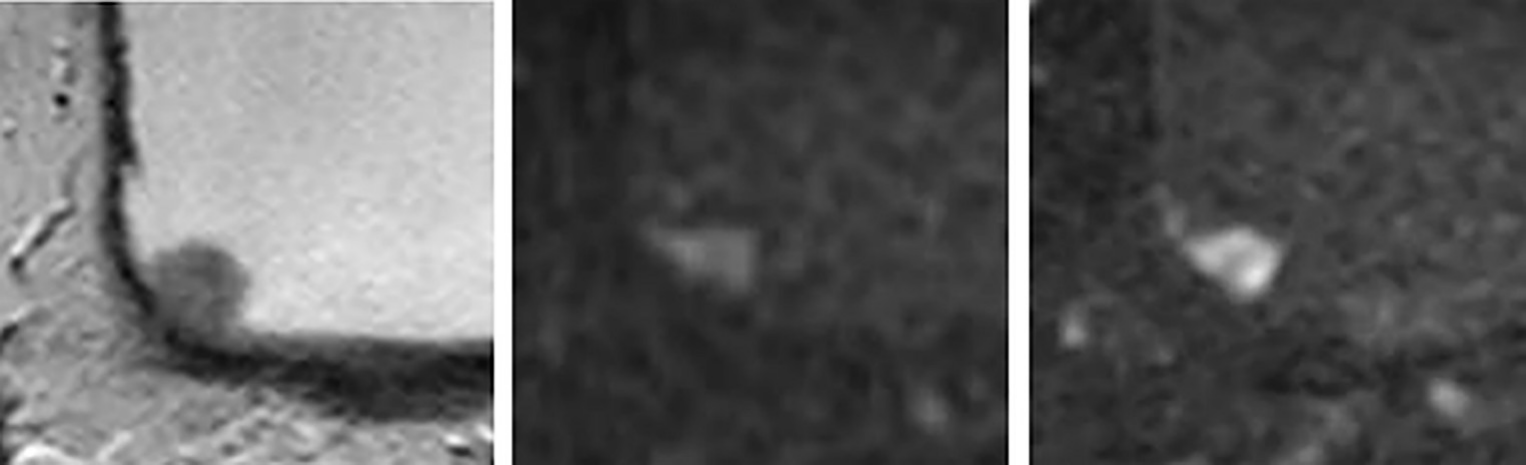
70-year-old man with the urothelial carcinoma of pTa on the right lateral to posterior wall of the bladder. (A) T2WI (B) fFOV DWI (C) Zoomed DWI. The tumor appeared as the lesion without tumor stalk in fFOV DWI, and VI-RADS score was 3 for both readers. In contrast, the tumor appeared as a papillary lesion with tumor stalk in Zoomed DWI, and VI-RADS score was 2 for both readers.
